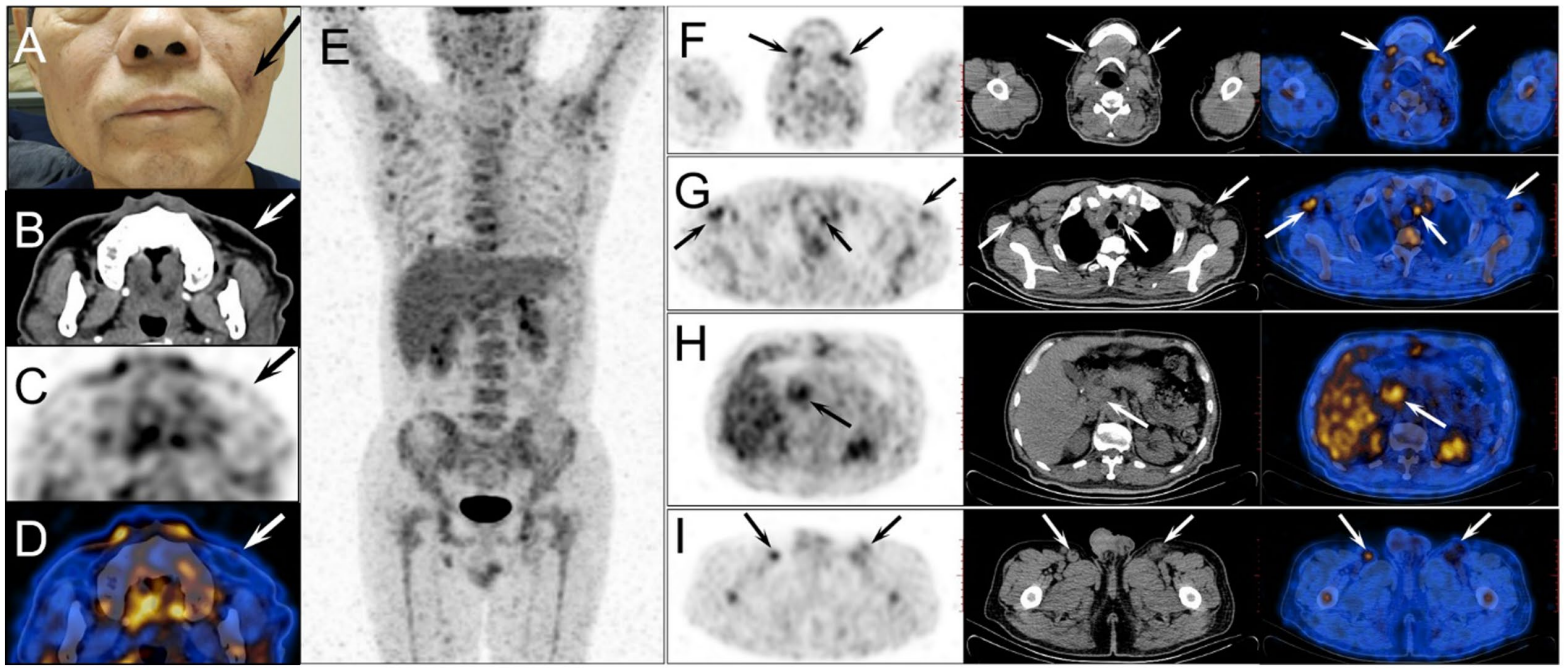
The physical examination revealed a brown rash on the patient’s left cheek [(A), arrow]. The axial image of head CT revealed slightly skin thickening in the left cheek [(B), arrow], with mild ^18^F-FDG avidity (SUVmax 1.1) on the corresponding PET [(C), arrow] and PET/CT fusion [(D), arrow] images. The body MIP image (E) demonstrated diffuse ^18^F-FDG uptake in bone marrow (SUVmax 4.0) and multiple metabolic foci as described in the following axial images. On the axial images of PET, CT and PET/CT fusion, multiple enlarged lymph nodes were found in bilateral submaxillary regions (F), bilateral axillas and mediastinum (G), hepatic hilum (H), and bilateral groins (I) (arrows), which exhibited varying ^18^F-FDG avidity with the maximal SUVmax of 4.2 in the left submaxillary lymph nodes.
